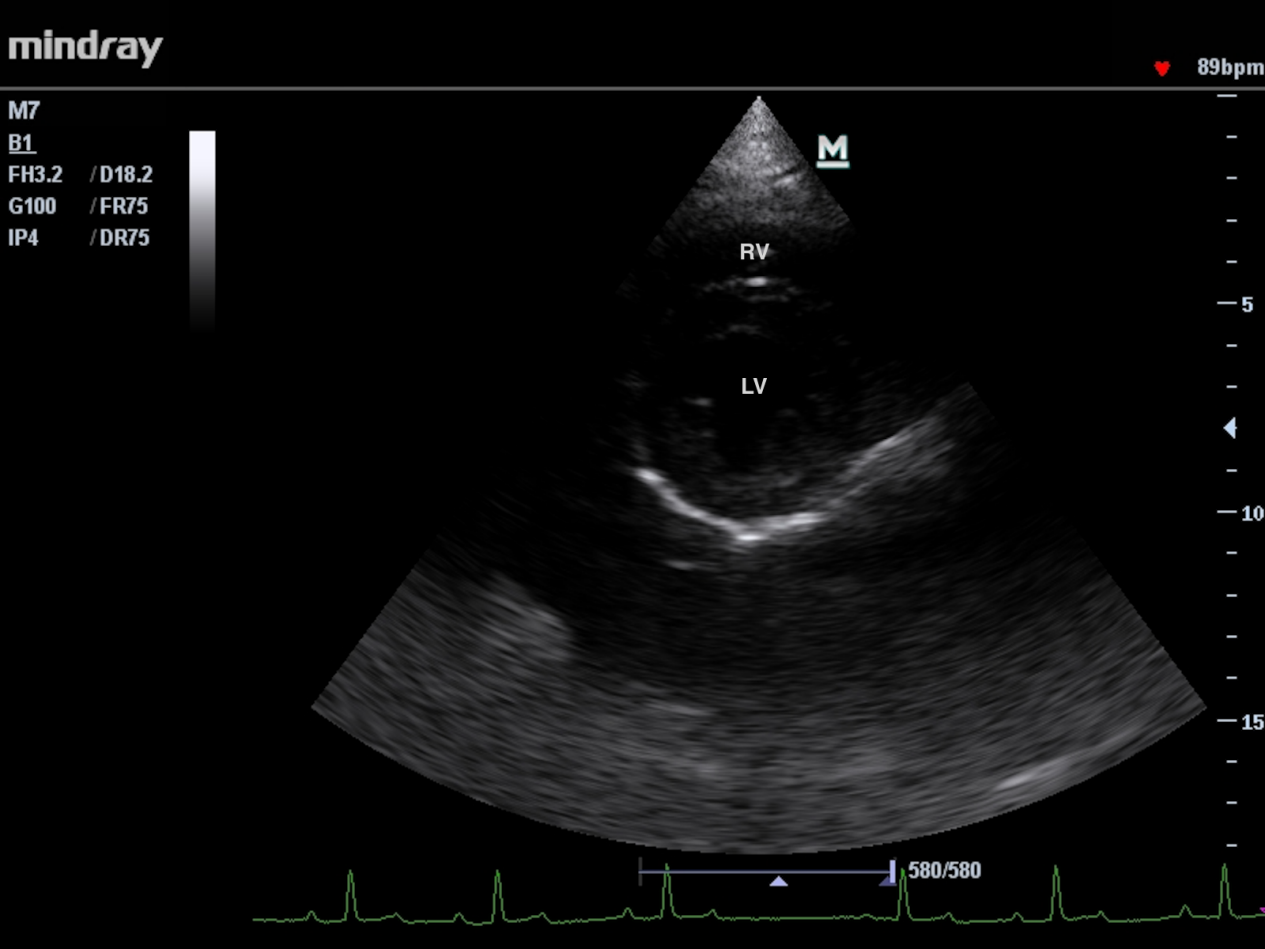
The right parasternal short-axis view of the left and right ventricles at the level of papillary muscles RV- right ventricle, LV- left ventricle.
